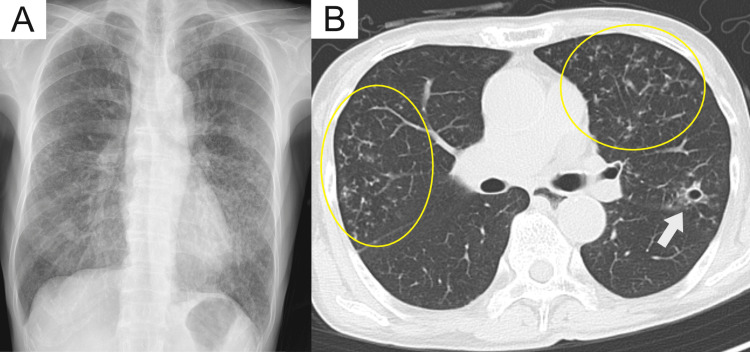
Chest radiograph and computed tomography A: Chest radiograph shows diffuse nodular opacities; B: Chest computed tomography shows a cavitary lesion (white arrows) in the left upper lobe and bilateral centrilobular bronchiolitis (yellow circles).
